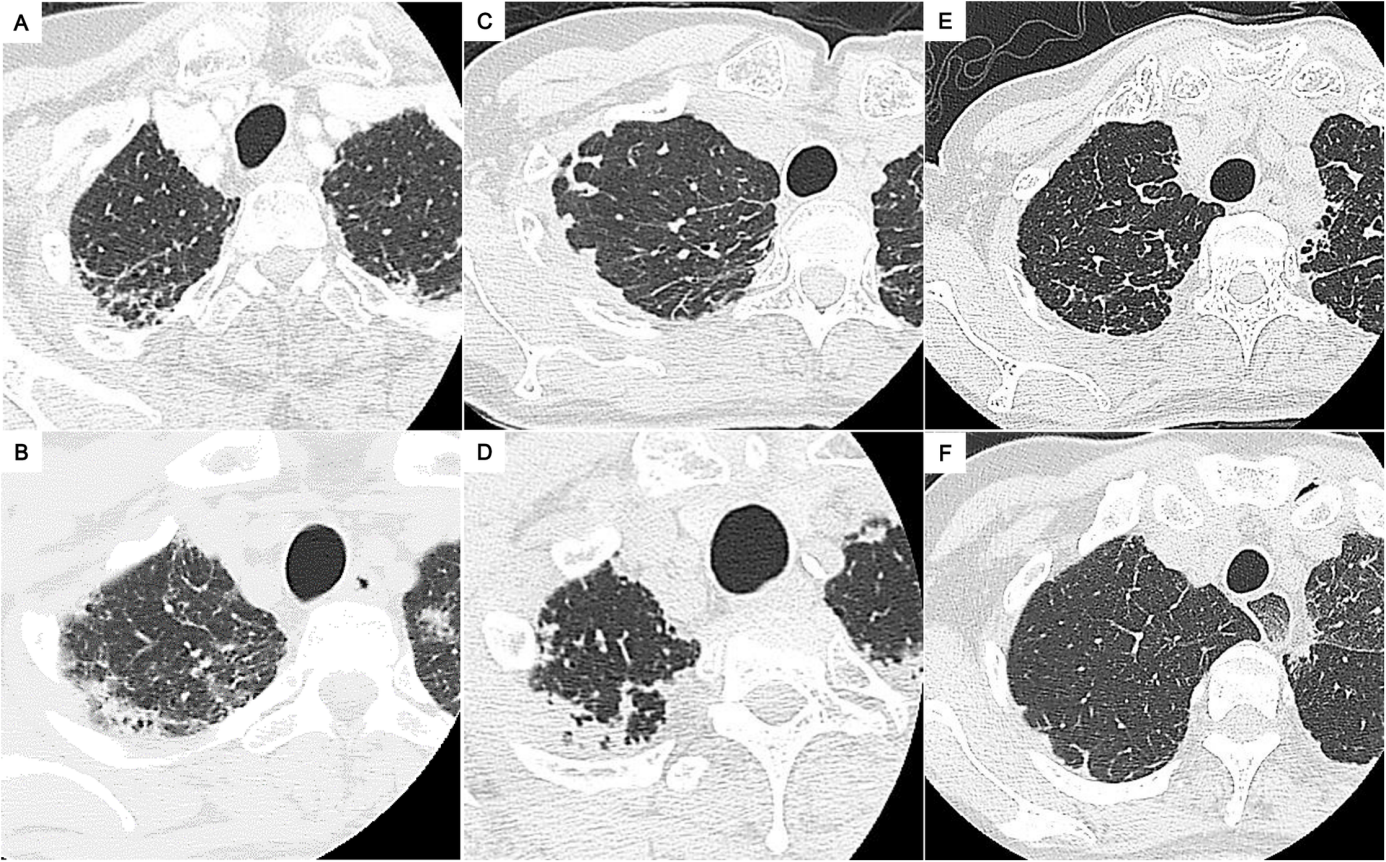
Representative chest computed tomography images of radiologic pleuroparenchymal fibroelastosis (PPFE)-like lesion. Images of the right lung apex in patient 2 (A), patient 9 (B), patient 11 (C), patient 16 (D), patient 17 (E), and patient 20 (F) are shown. The images (E) and (F) reveal minimal cases of PPFE-like lesion we defined. Each patient number is listed in S1 Table.
